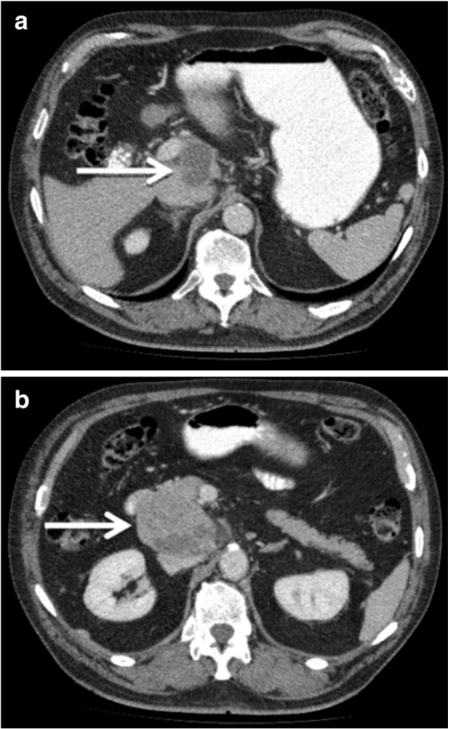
CT scan showing a large hypodense mass between the liver and the pancreas head (2a and 2b)
